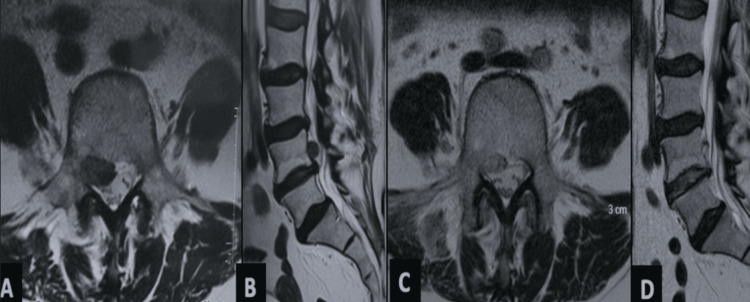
(A, B) Preoperative MRI of a 58-year-old male showing a high upmigrated right-sided disc prolapse reaching the L4 pedicle, Michigan State University type 2AB. (C, D) Postoperative MRI following the inside-out technique under local anesthesia without foraminoplasty, demonstrating adequate decompression with a residual minute fragment and no surgical footprints. The patient had an uneventful, symptom-free follow-up.
